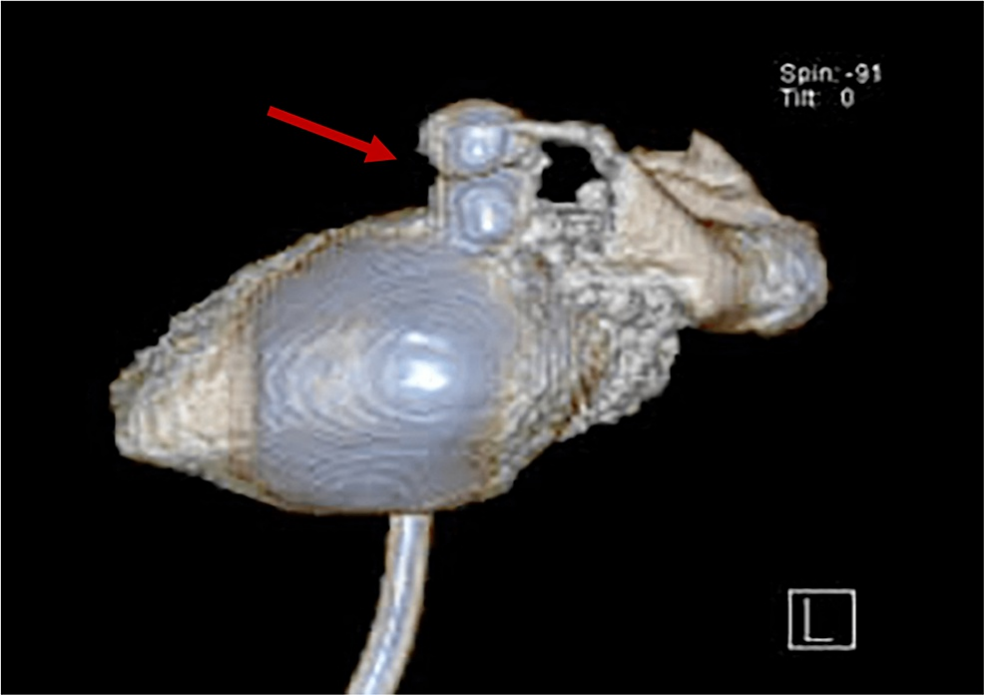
CT scan reconstruction showing the bladder diverticulum rupture.
